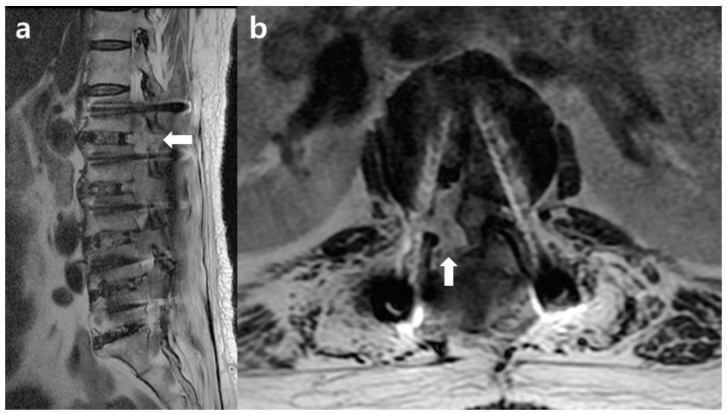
Postoperative lumbar MRI showed compressive myelopathy by extensive epidural hematoma from L1 to L3. (a) Sagittal; (b) axial cut on T2WI, MRI = magnetic resonance imaging. White arrows point to epidural hematoma.
